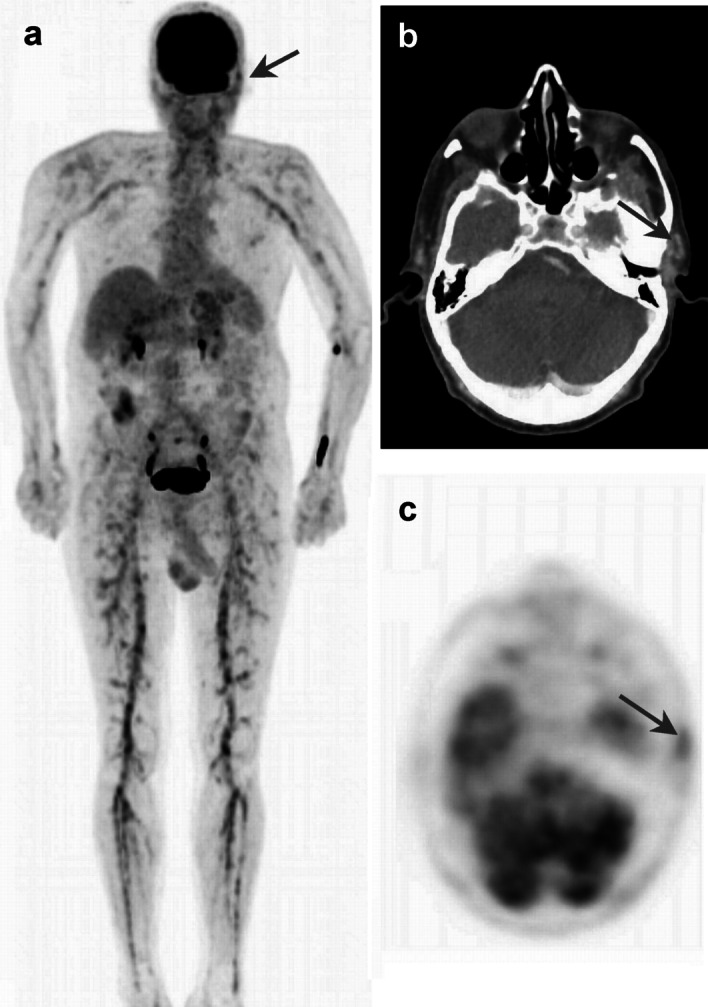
Total body [18F]FDG PET/CT was performed; coronal maximal intensity projection (MIP) image (a) showed increased FDG uptake of moderate-intensity along medium-sized extra-cranial (maxillary, and superficial temporal arteries), and cranial vessels; corresponding axial contrast-enhanced CT and [18F]FDG-PET (b, c) showed involvement of the left temporal artery with pathological tracer uptake (arrow), indicating active disease
